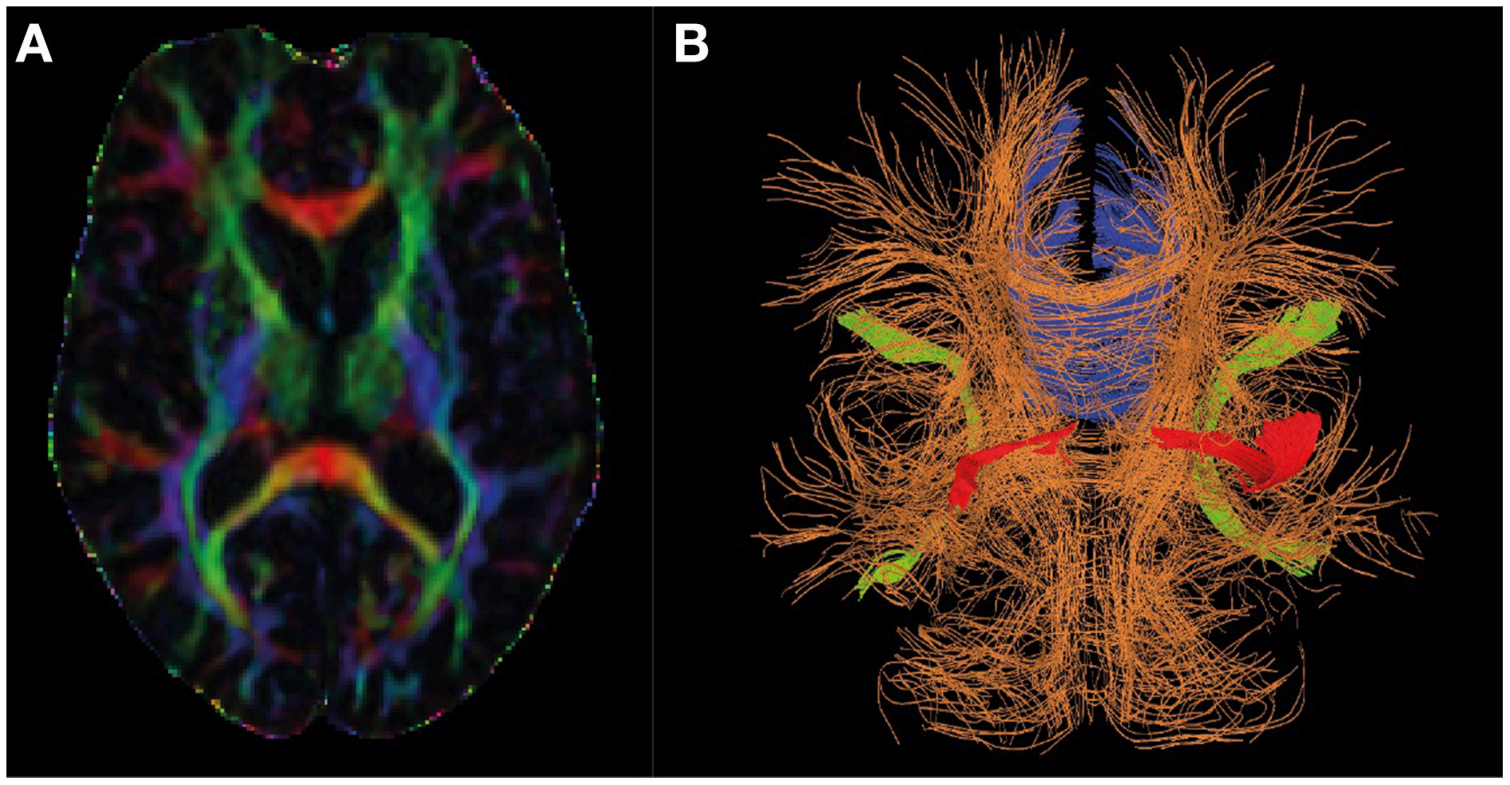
Diffusion tensor MRI in Alzheimer's research. Panel (A) shows the directional information encoded by diffusion tensor imaging by color (red: left-right, green: anterior-posterior, blue: head-foot) and fractional anisotropy (high intensity implies high fractional anisotropy). Panel (B) shows how the directional information may be represented in a deterministic tractography analysis, and shows the regions consistently identified and changing in AD (Blue: Corpus callosum, red: uncinate fasciculus, green: superior lateral fasciculus).
